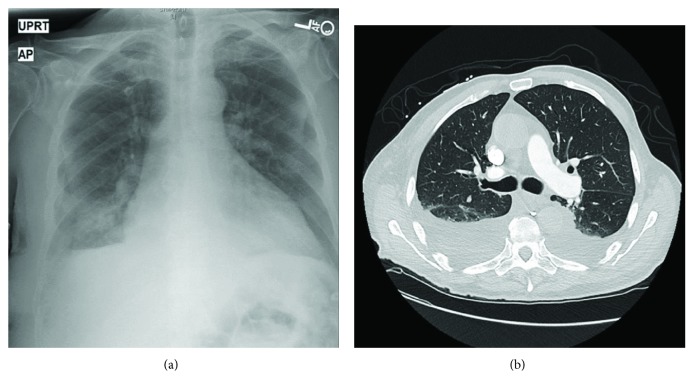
(a) CXR showed cardiomegaly with pulmonary congestion and bilateral pleural effusion. (b) CT chest with contrast showed moderately large bilateral pleural effusions, right greater than left, with cardiomegaly and reflux of IV contrast into the IVC, consistent with cardiogenic pulmonary vascular congestion.
